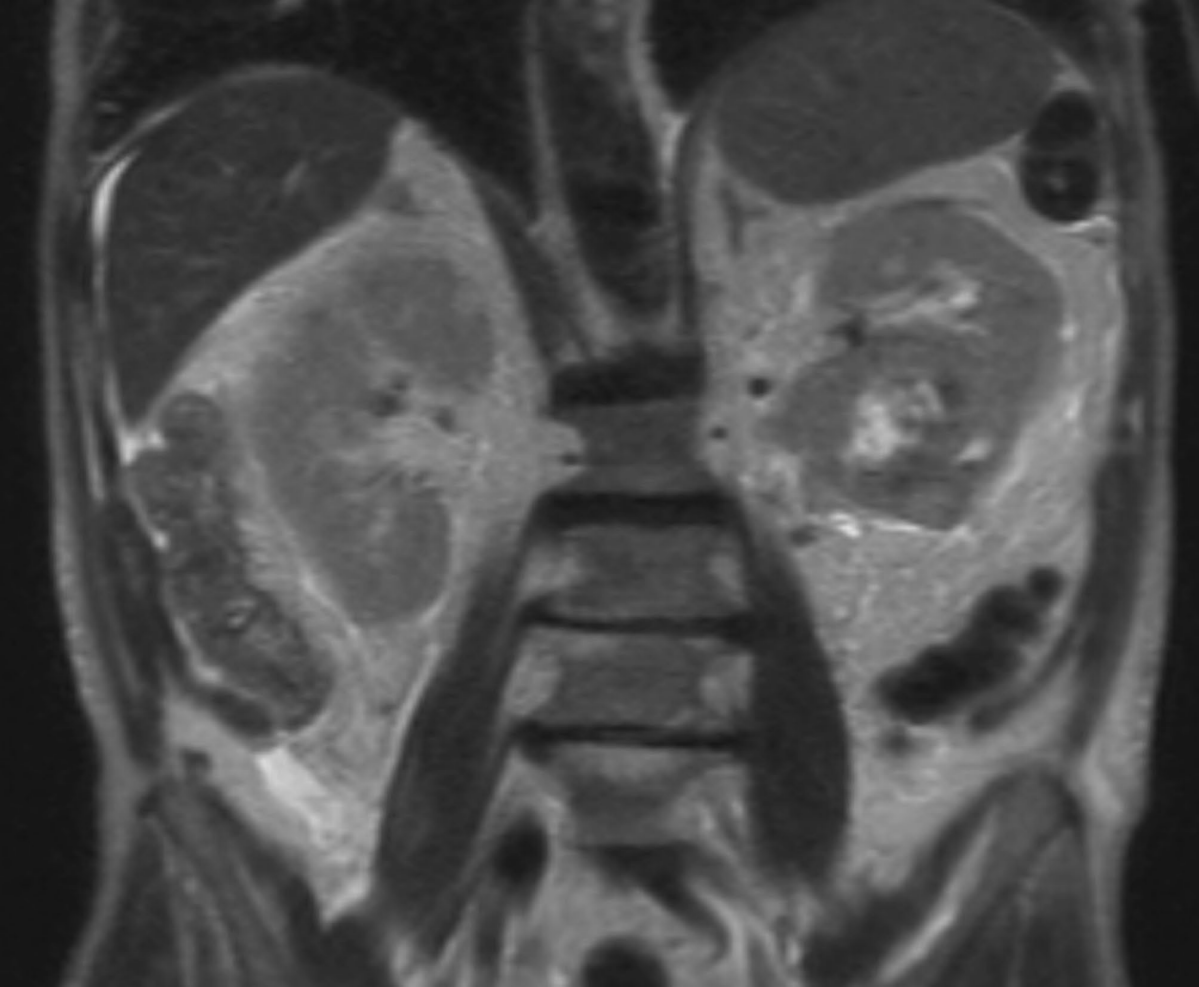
Magnetic resonance imaging (MRI) of the abdomen and pelvis with and without contrast revealing a 7.1-cm left lower pole renal mass.
